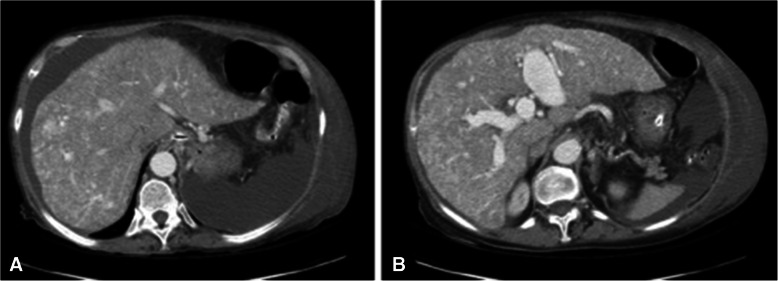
A and B Axial CT images with contrast of a 77-year-old female with HHT and portal hypertension showing cirrhotic features due to pseudocirrhosis. Findings consistent with HHT and portal hypertension including ascites, hypertrophy of the caudate lobe, portal venous enlargement, and varices
